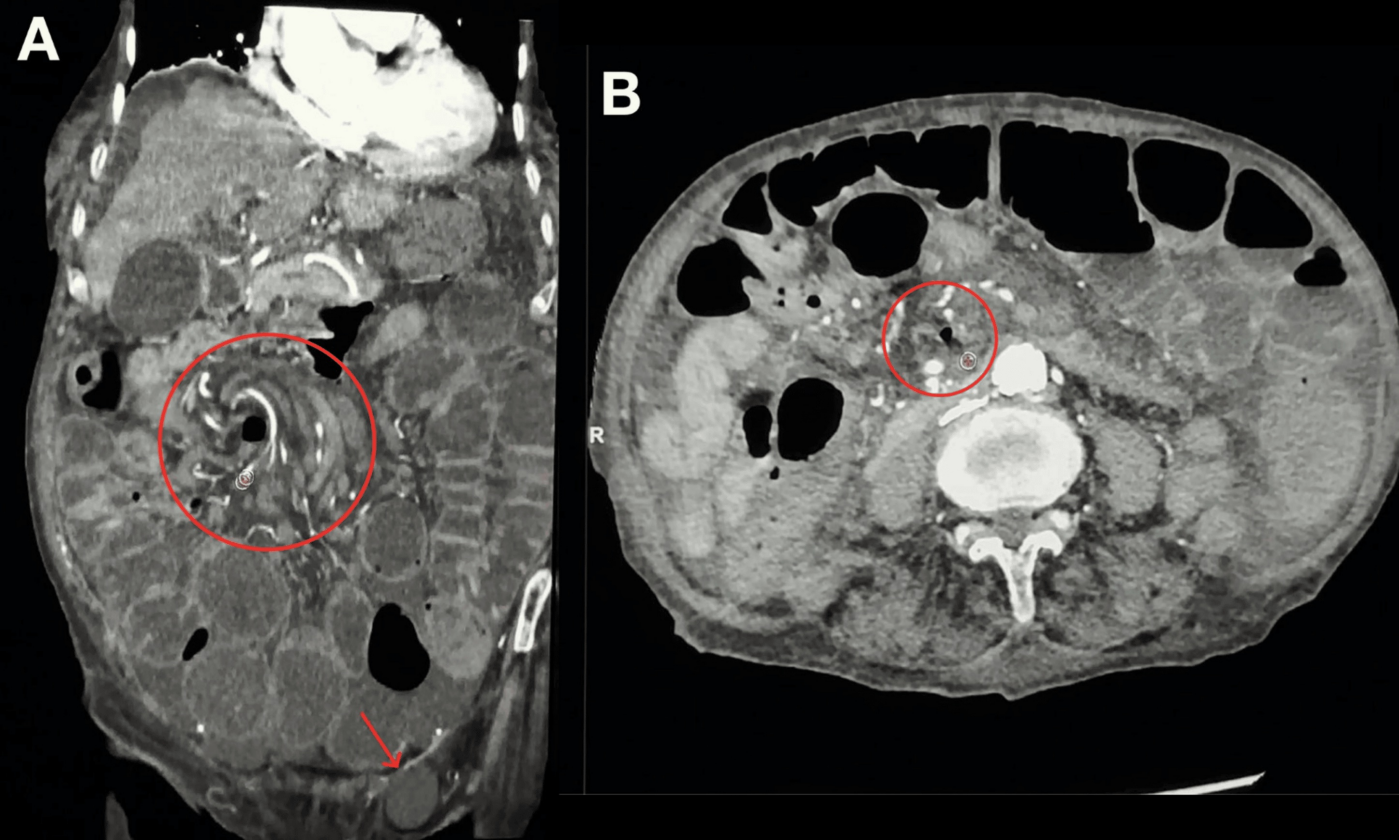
Contrast-enhanced CT images demonstrating the whirlpool sign (A) Coronal CT image demonstrating the whirlpool sign (circle) with an associated small left femoral hernia (arrow). (B) Axial contrast-enhanced CT image demonstrating the whirlpool sign (circle).
